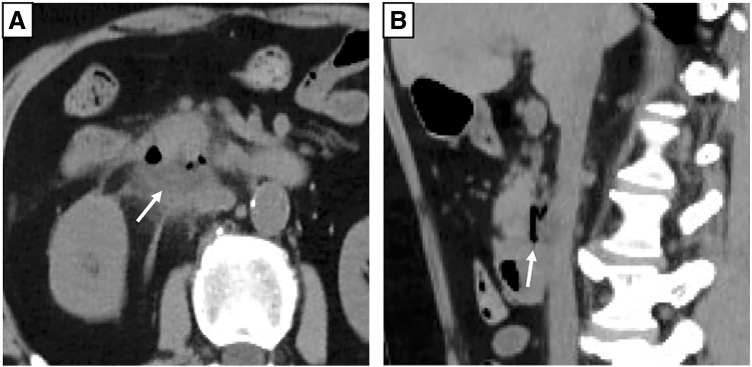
CT scan from previous hospitalization. A white arrow in both axial (A) and sagittal (B) CT scans indicates dorsal duodenal purulent effusion and internal air. CT, computed tomography
